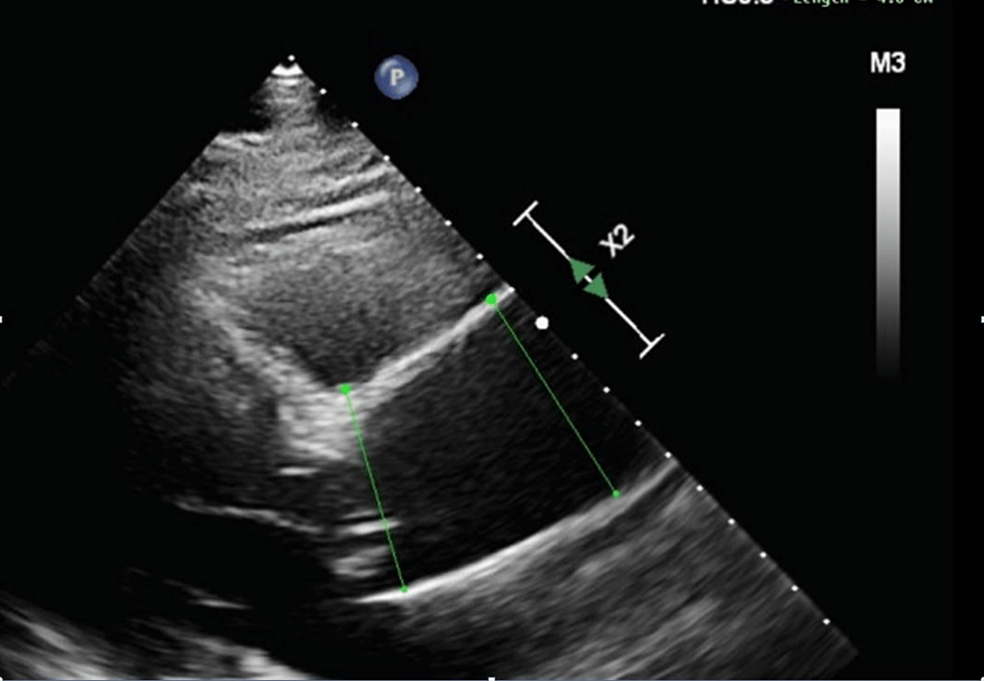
Echocardiogram; dilated ascending aorta
